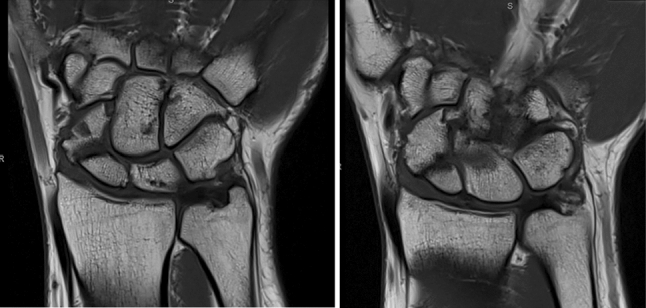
Preoperative MRI scan (coronar T1)
